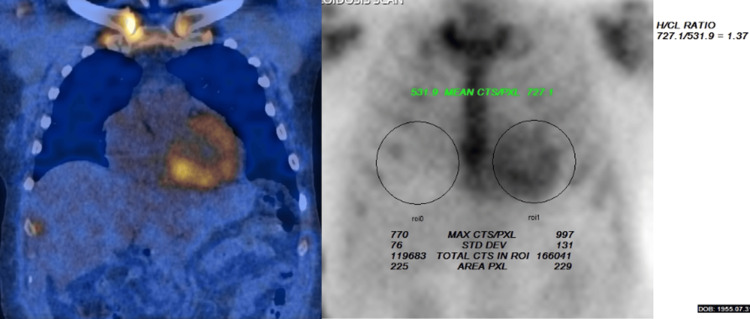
Visual semiquantitative radiotracer uptake in the myocardium from the SPECT-CT is more than rib uptake (grade 3). Quantitatively, the heart to contralateral lung ratio (H/CL) is 1.37
